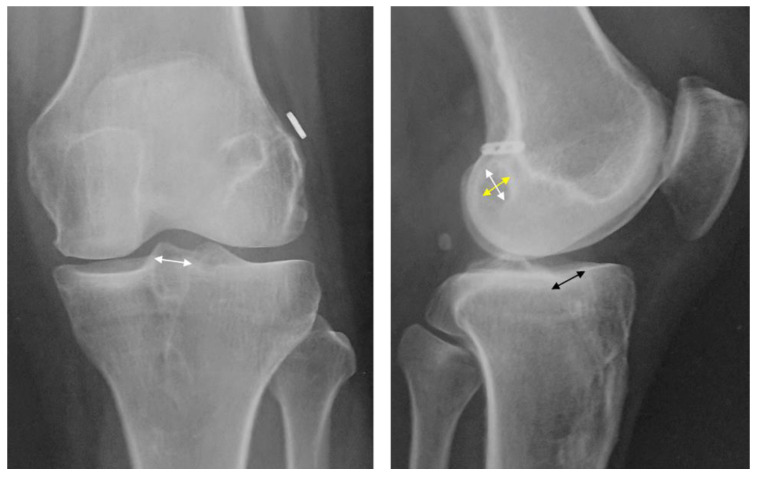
Radiographic findings at the final observation. Bone tunnel enlargement evaluation in the X-ray front view (left), the medial-lateral diameter of the tibial bone tunnels at the aperture sites that were between the inner aspect of the sclerotic tunnel wall was measured (white arrow). In the lateral radiograph (right), the anterior-posterior diameter (yellow arrow), proximal-distal diameter (white arrow) of the femoral bone tunnel, and anterior-posterior diameter of the tibial bone tunnel (black arrow) at the aperture sites that were between the inner aspect of the sclerotic tunnel wall were measured.
